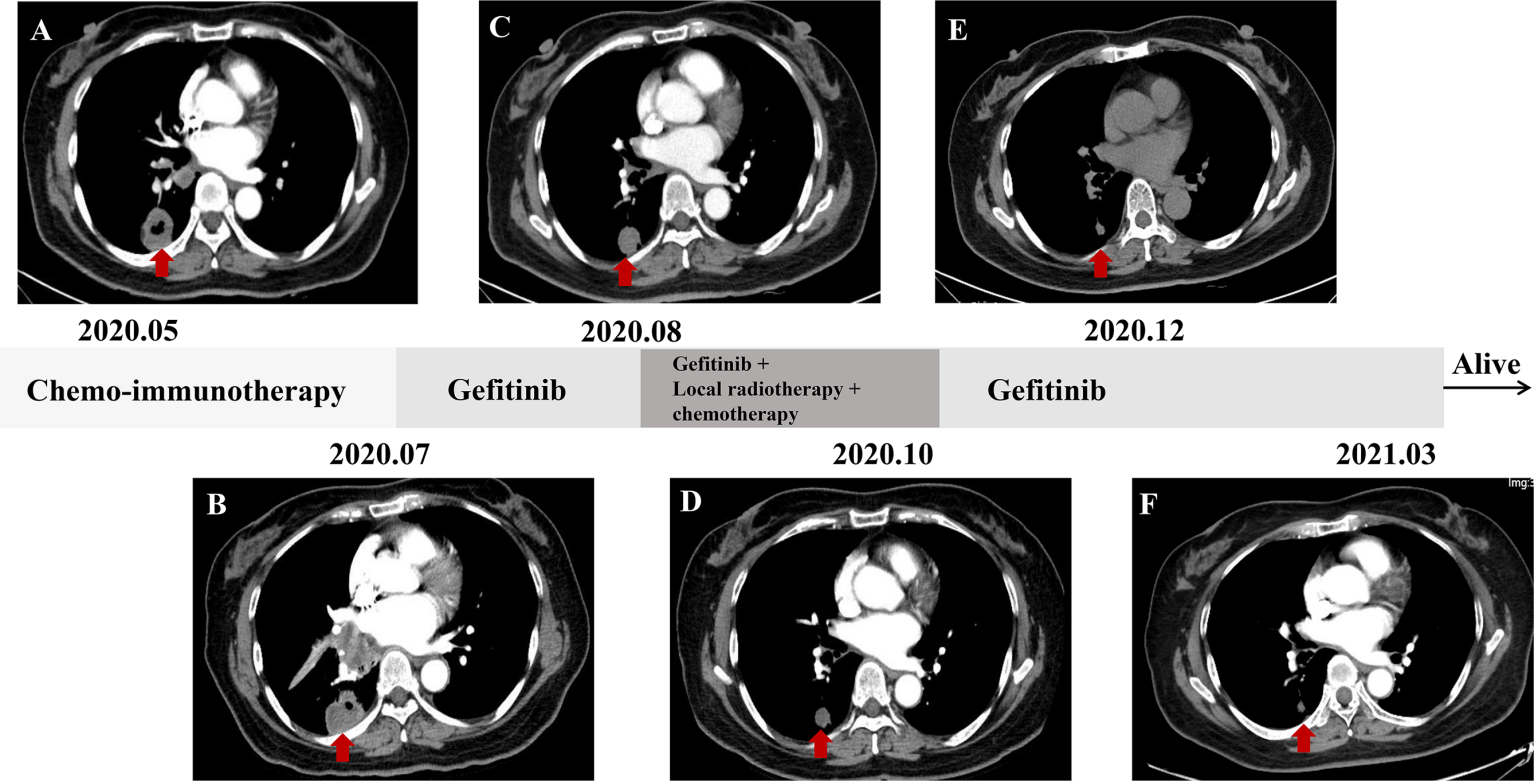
Chest CT scanning of the patient. (A) baseline; (B) after one cycle of neoadjuvant immuno-chemotherapy (albumin-bound paclitaxel + carboplatin + nivolumab 240 mg); (C–F) chest images at indicated time points.
